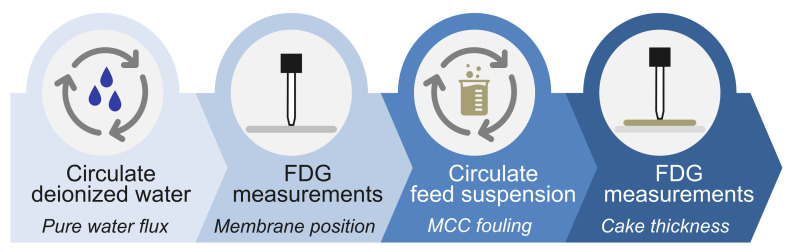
Process flow of the cross-flow MF of the MCC suspensions and FDG measurements.
